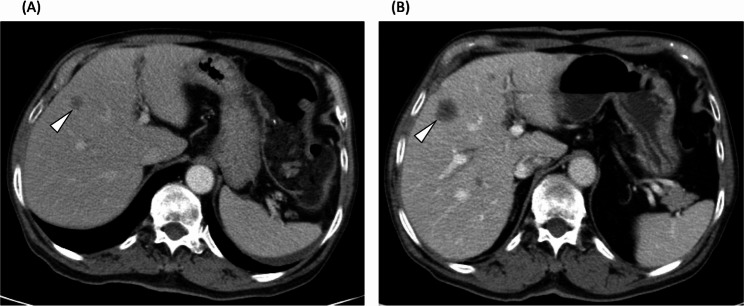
A 72-year-old with moderately-differentiated adenocarcinoma of sigmoid colon with liver metastasis. Before initiation of bevacizumab-based chemotherapy (A), CT scan showed a 1.1-cm metastatic lesion (arrowhead) in the segment 5/8 of liver. After three months of treatment with bevacizumab-based chemotherapy, CT scan (B) demonstrated increased size (2.2 cm) of this metastatic lesion (arrowhead) with indistinct liver-tumor surface. RD-MR defined this patient as a non-responder. In ResNet-MR, this patient was classified as a responder. The patient survived for 28 months after initiation of bevacizumab-based chemotherapy and was alive on the date of final censoring
